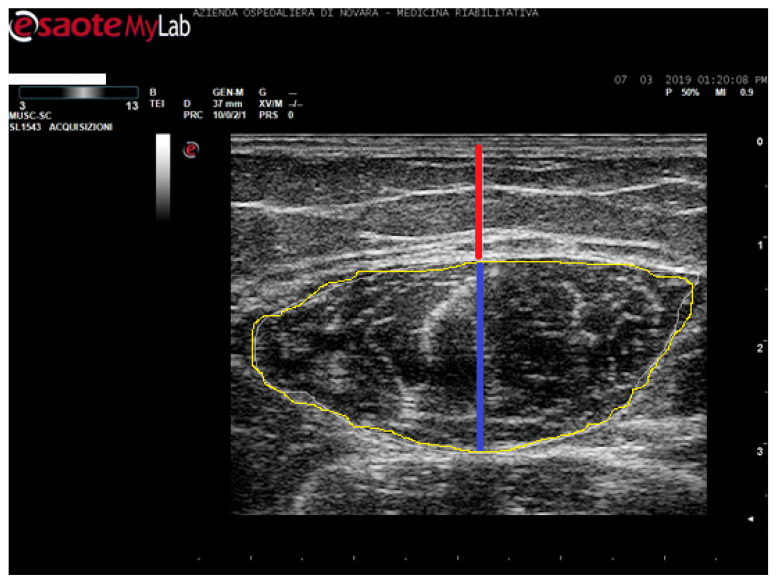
Real ultrasound images of RF. MD measurement is shown in red; MT measurement is shown in blue; CSA measurement is shown in yellow. MEI is calculated on the area circled in yellow using ImageJ.
